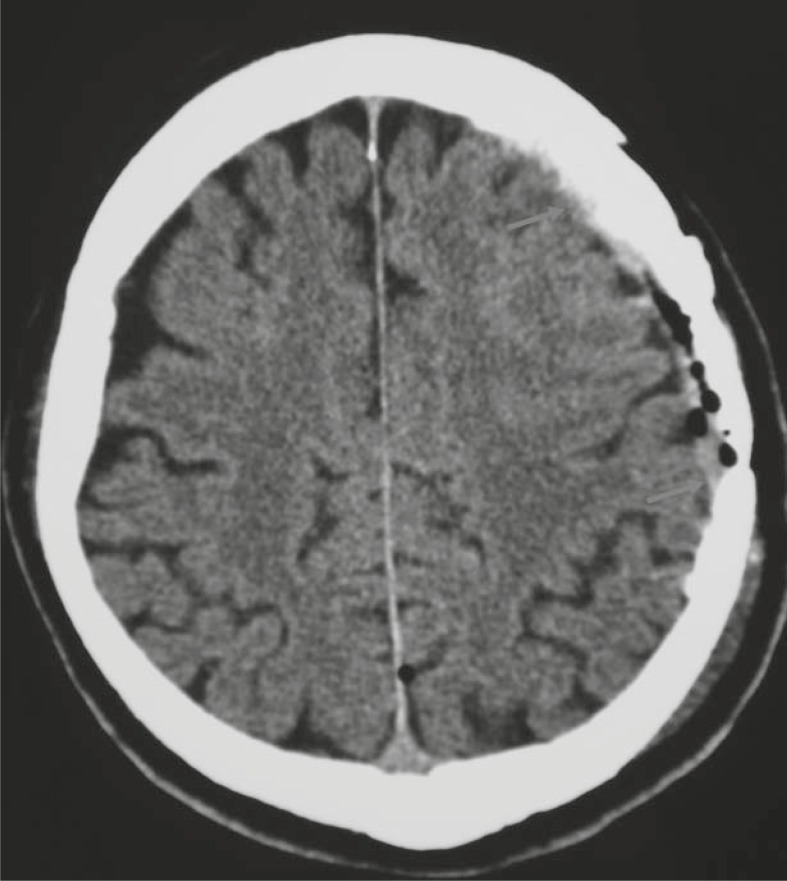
The axial view of the postoperative CT scan with the artificial bone (arrows) covering slightly oedematous brain tissue.
